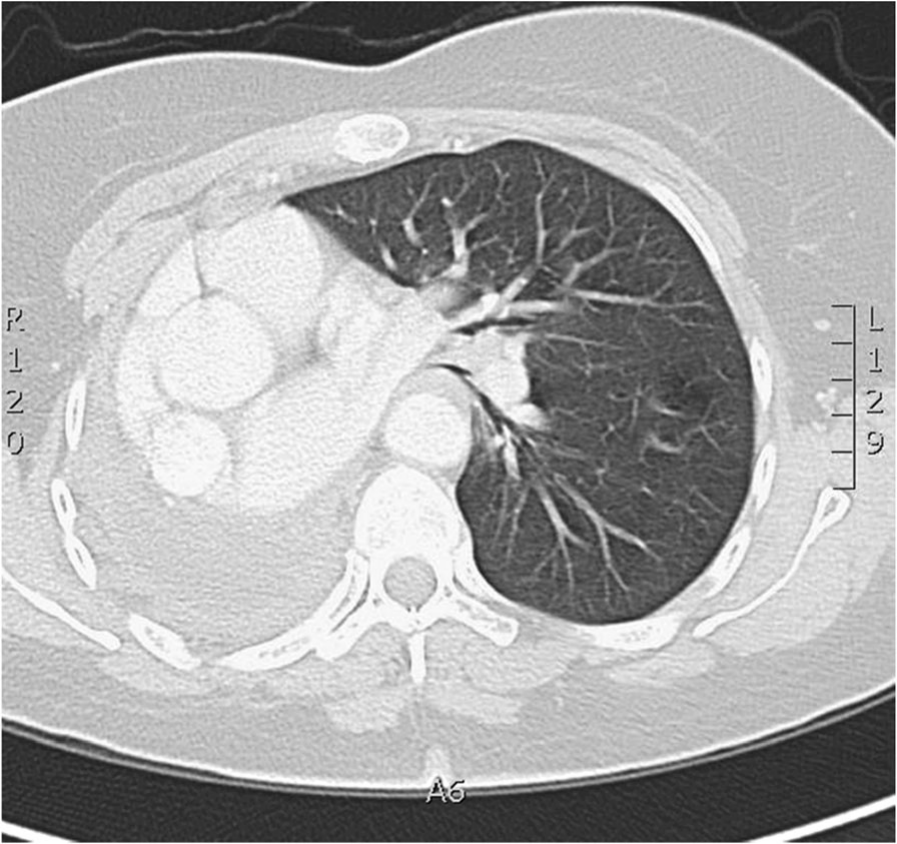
Follow up CT chest after 6 months shows Right-sided pneumectomy with slightly hyperinflated contralateral lung
